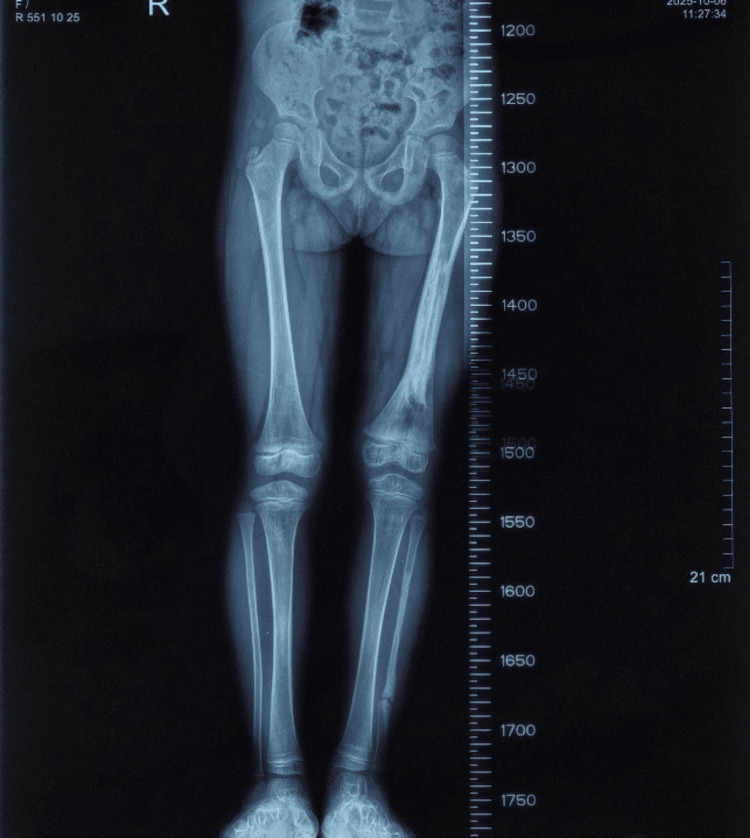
Full length anteroposterior (AP) view radiograph showing remodelling of the femur over the graft at eight months.
